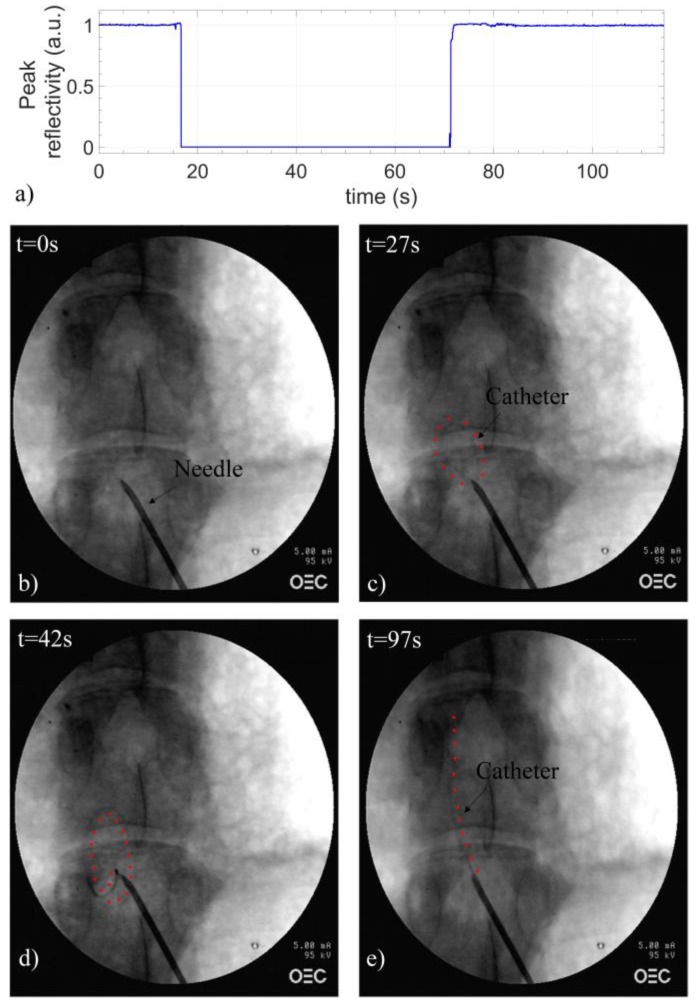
(a) Peak reflectivity as a function of time recorded during the insertion of the sensorized EC in L5/L6 space; X-ray images of the catheter (b) in the epidural needle and in different positions inside the ES: (c) curved, (d) coiled and (e) straight. Red dots, placed at the catheter’s side, highlight its trajectory.
